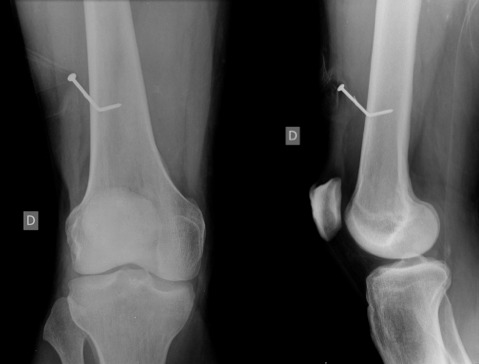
Radiographie du genou montrant un clou intraosseux
